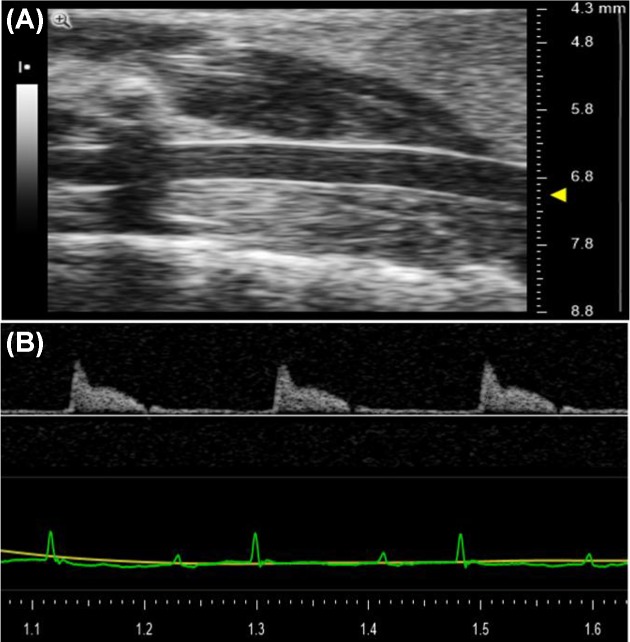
Echocardiography and Doppler echocardiography image display (A) B-mode (2D) echocardiographic image of the RCCA. Arrow on the right side indicates the RCCA. (B) Doppler echocardiographic image of RCCA FL.
